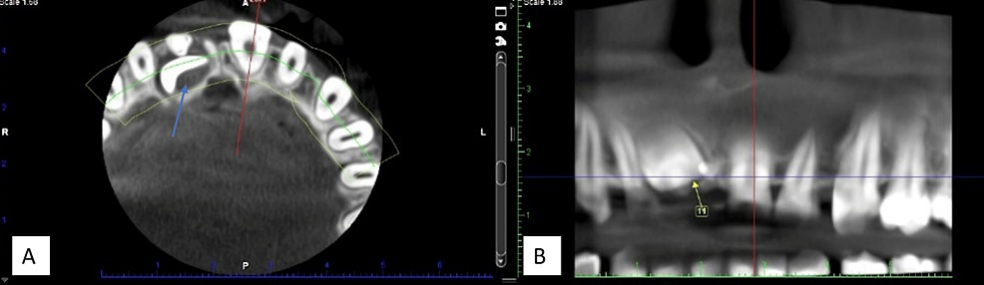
(A) CBCT Image showing supernumerary teeth with the impacted central incisor in blue arrow on axial view (B) Panoramic view showing the distance of the impacted central incisor from the alveolar crest CBCT: Cone-beam CT
